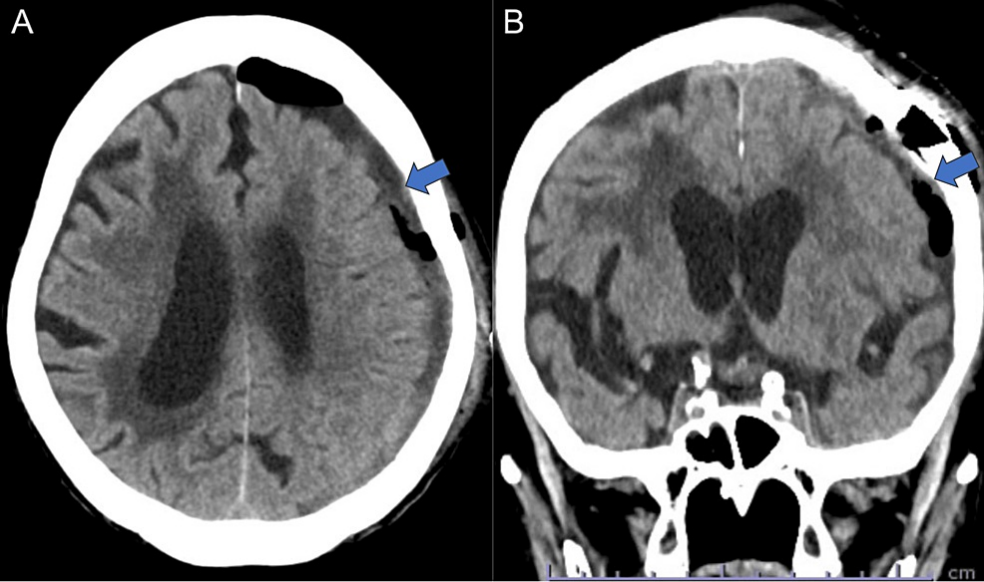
Postoperative computed tomography imaging Axial computed tomography (CT) images 1 day after endoscopic surgery showing gross total hematoma evacuation (blue arrows).
